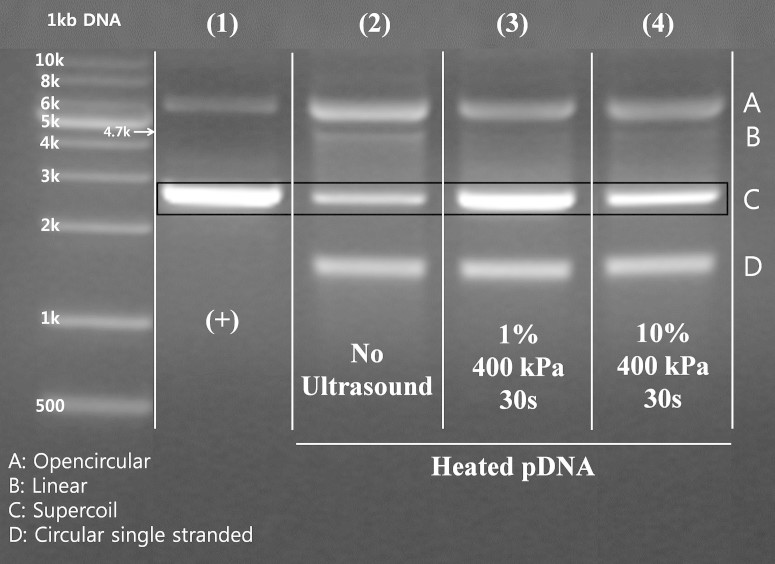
The results of electrophoresis in comparison to normal pEGFP, heated pEGFP, and heated pEGFP after ultrasound sonication. lane(1): normal pEGFP vector, lane(2): heated pEGFP vector, lane(3): heated pEGFP vector which is sonicated by ultrasound (pressure: 400 kPa, duty percentage: 1%, sonication time: 30 s), lane(4): heated pEGFP vector which is sonicated by ultrasound (pressure: 400 kPa, duty percentage: 10%, sonication time: 30 s).
